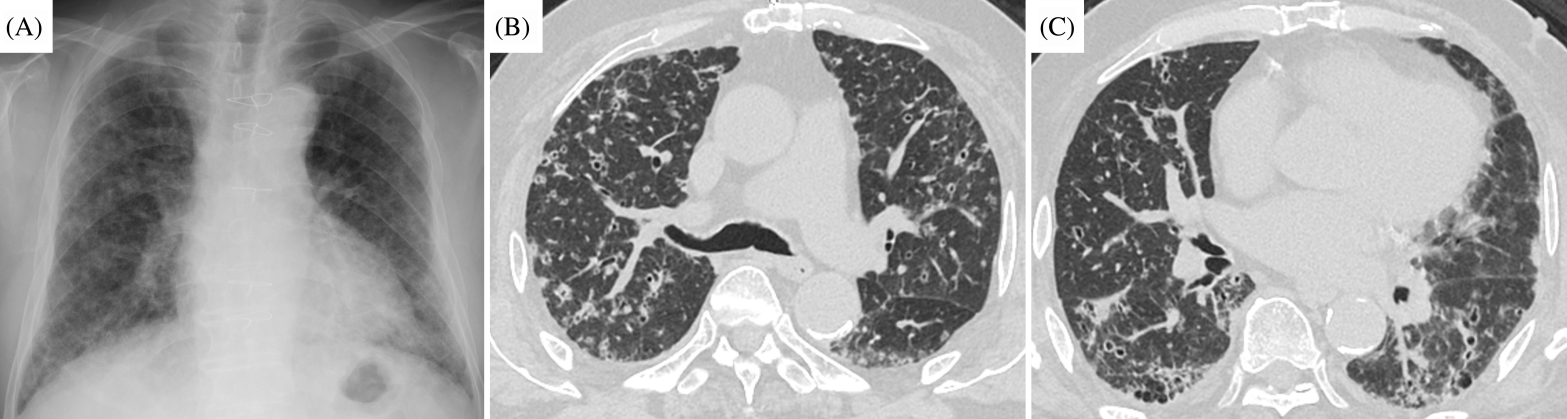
Chest radiograph and Chest computed tomography of the first examination. (A) Chest radiograph showing diffuse bilateral reticular shadows and multiple nodules. (B, C) Chest computed tomography (CT) shows multiple diffuse cavities bilaterally, multiple micronodules (<5 mm), and subpleural reticular shadows in the bilateral lower lobes.
